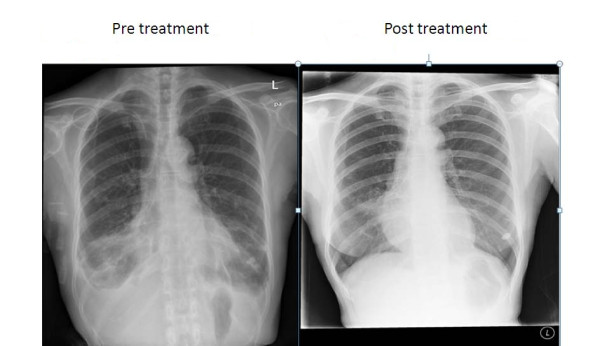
Pre-treatment and post-treatment chest radiographs. After two months of antifungal therapy, there was complete resolution of the bilateral pleural effusion with significant improvement in the bibasal consolidation.
